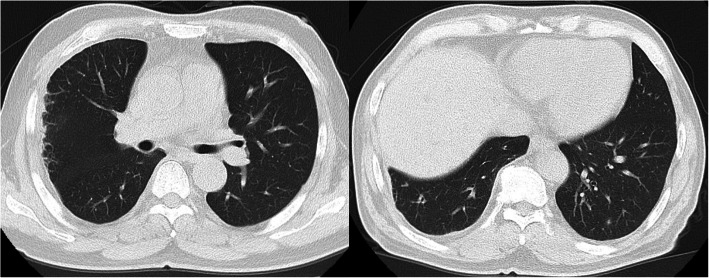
Chest computed tomography scan revealing slight right-sided pleural effusion and pulmonary infection. Date: November 16, 2018
